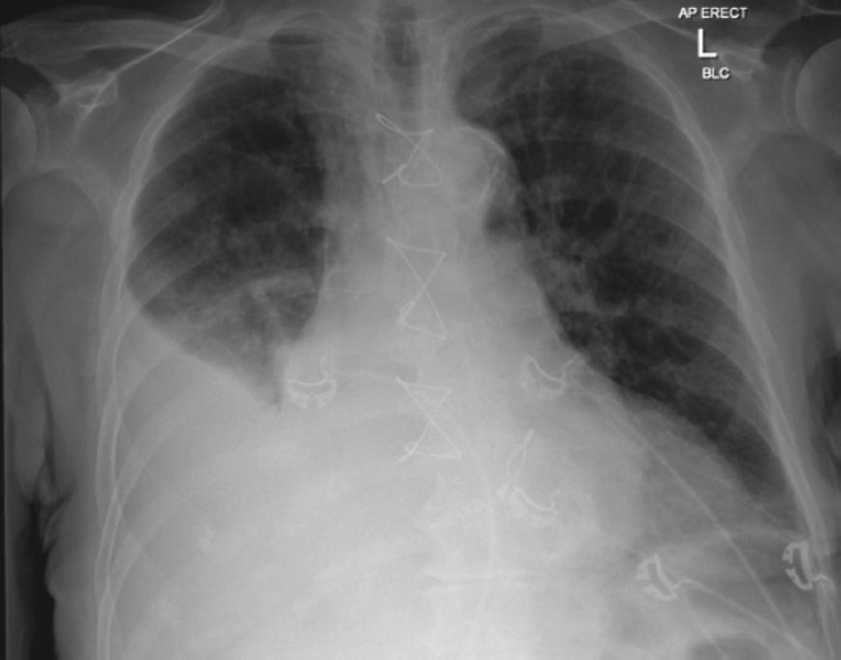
Chest radiograph on presentation showing evidence of right-sided pleural effusion
